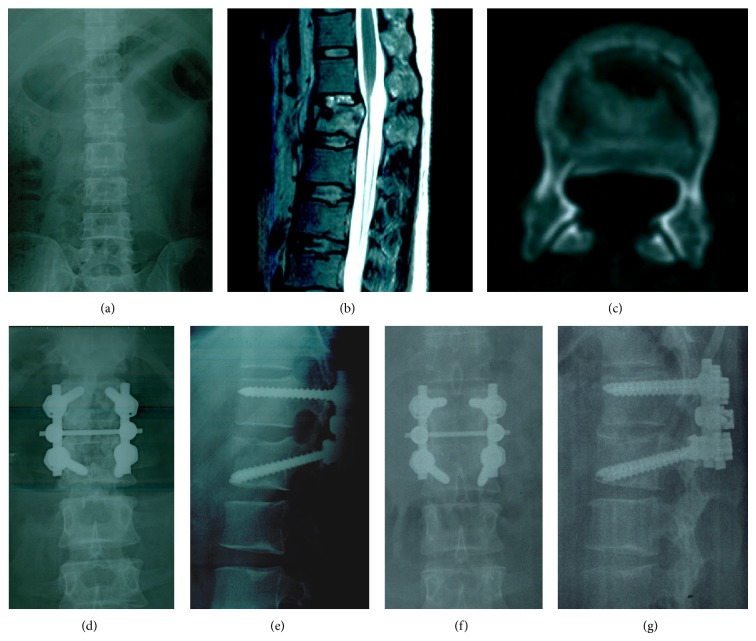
(a) Preoperative anterior-posterior X-ray; (b) preoperative MRI sagittal; (c) preoperative CT cross section; (d) and (e) postoperative radiographs; swing the tail of polyaxial pedicle screws caudally to coincide with the normal curve of spine; (f) and (g) radiographs after 61 months.
